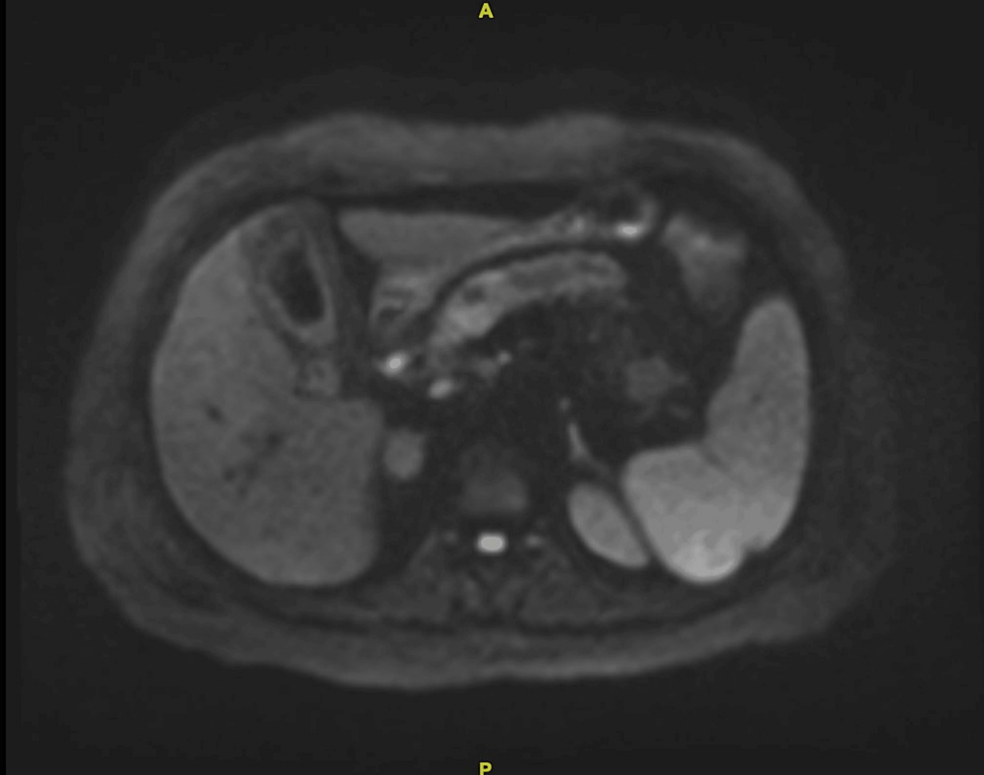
Axial MRI for patient 2 Axial MRI showing the body and tail of the pancreas with dilated ducts but no mass.
